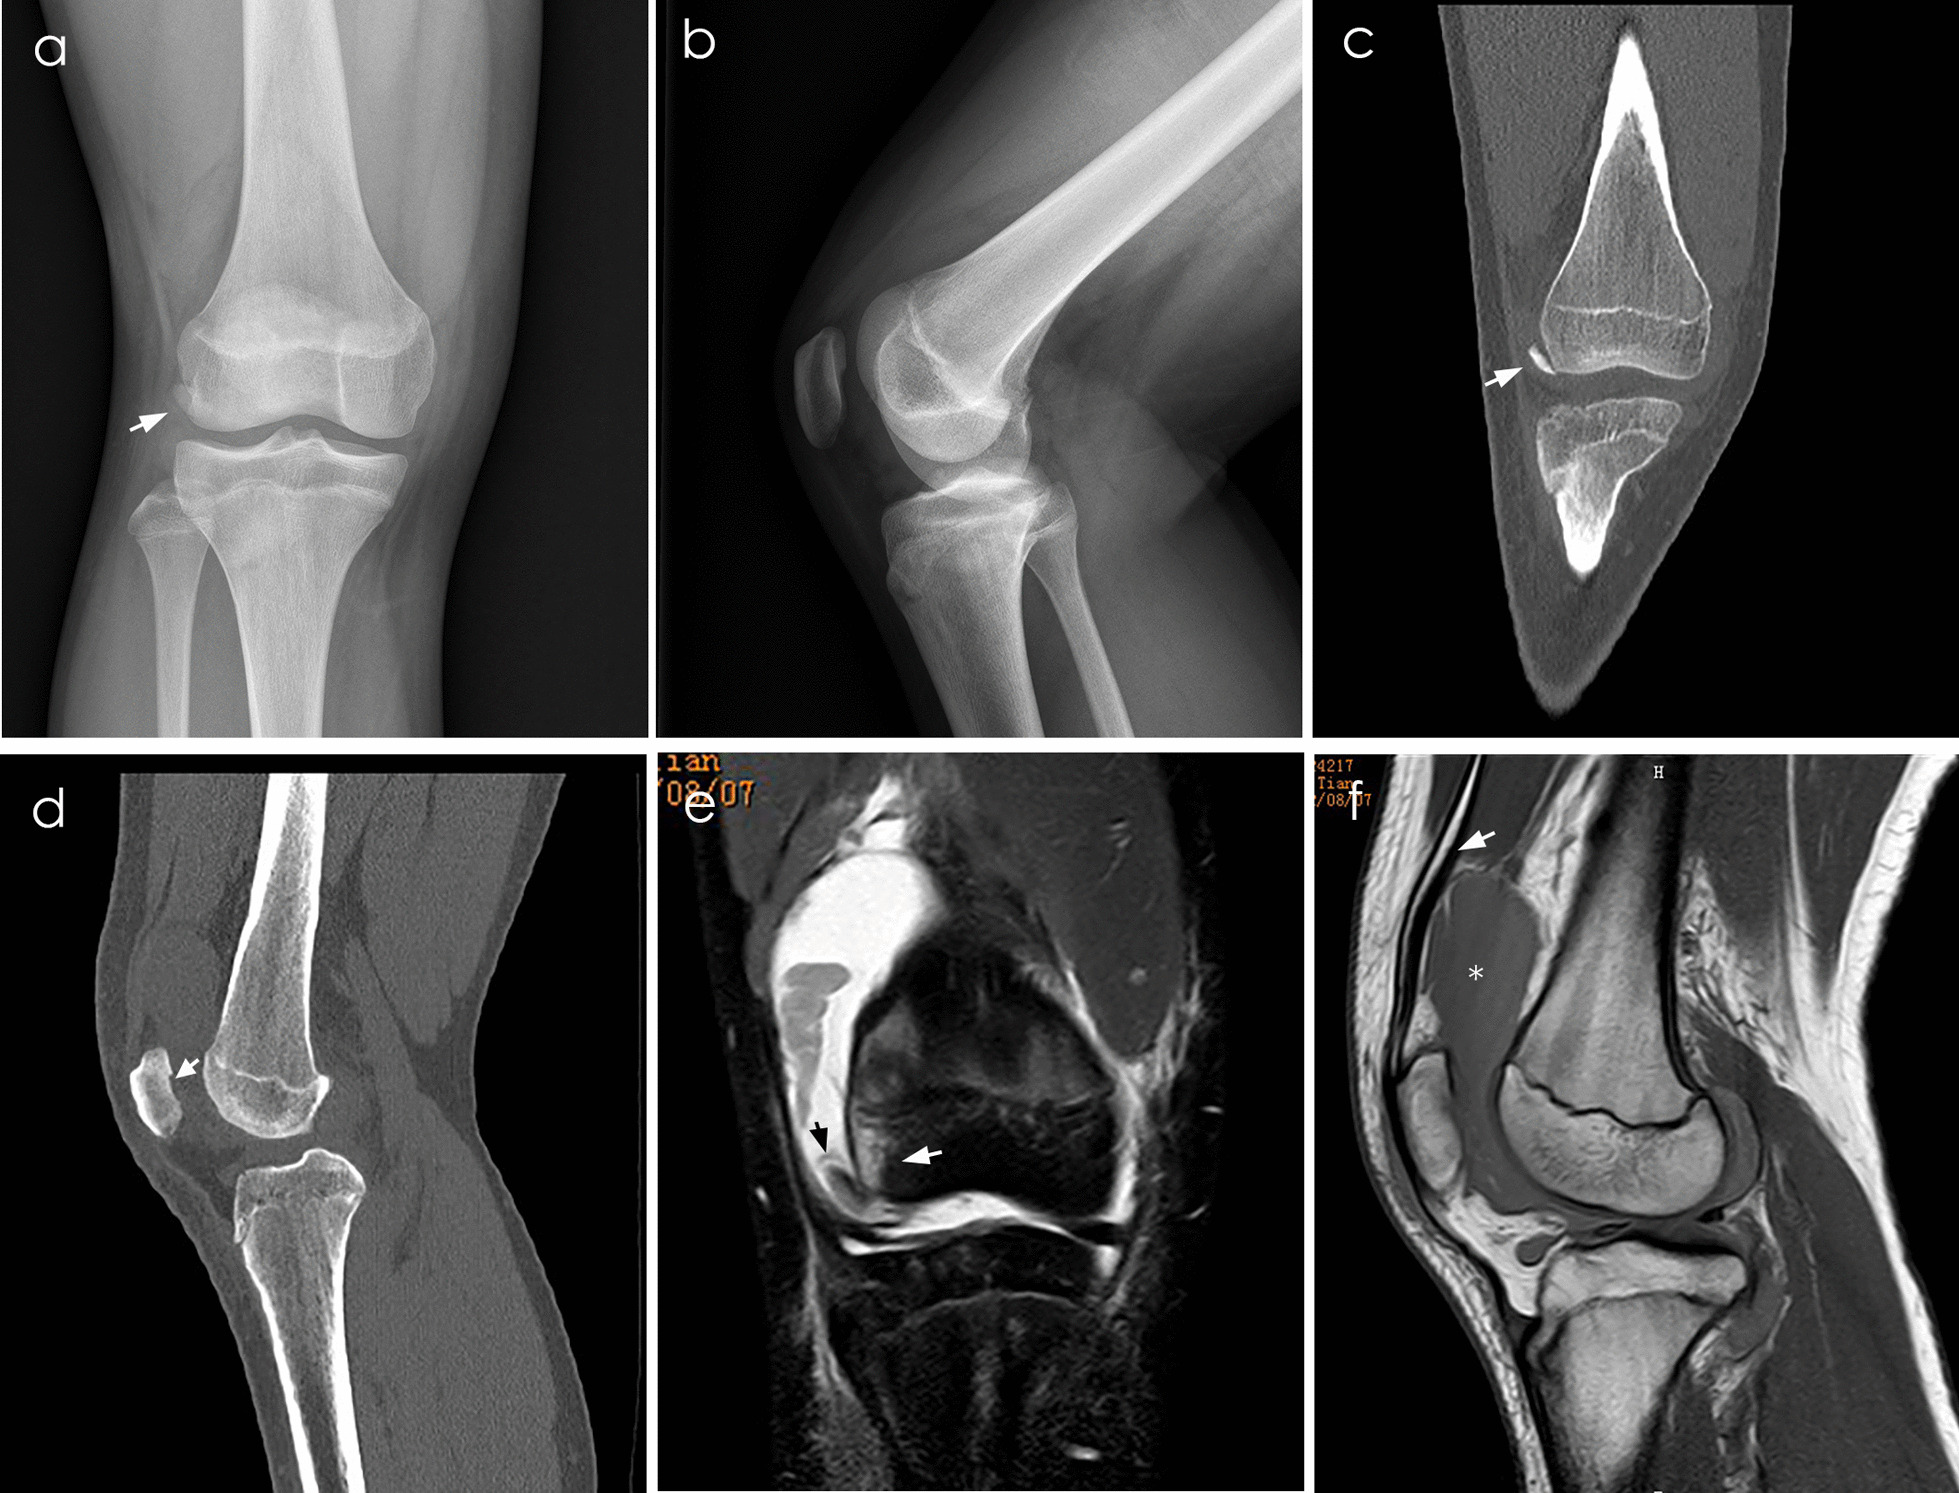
Plain radiograph, CT, and MRI of a 14-year-old boy with right traumatic patellar dislocation. a Anteroposterior knee radiograph shows the fracture fragment overlapping with the femoral lateral condyle, which resulted in a missed diagnosis. b Lateral knee radiograph. c Coronal view of CT showing the fracture fragment clinging to the femoral lateral condyle (white arrowhead). d Sagittal view of CT showing patella articular defect lesion (white arrowhead). e T2-weighted MRI showing fracture fragments in the lateral sulcus of the knee (black arrowhead) and contusion of the lateral condyle of the femur (white arrowhead). f T1-weighted MRI showing obvious SP pouch effusion (star) and quadriceps tendon (white arrowhead)
